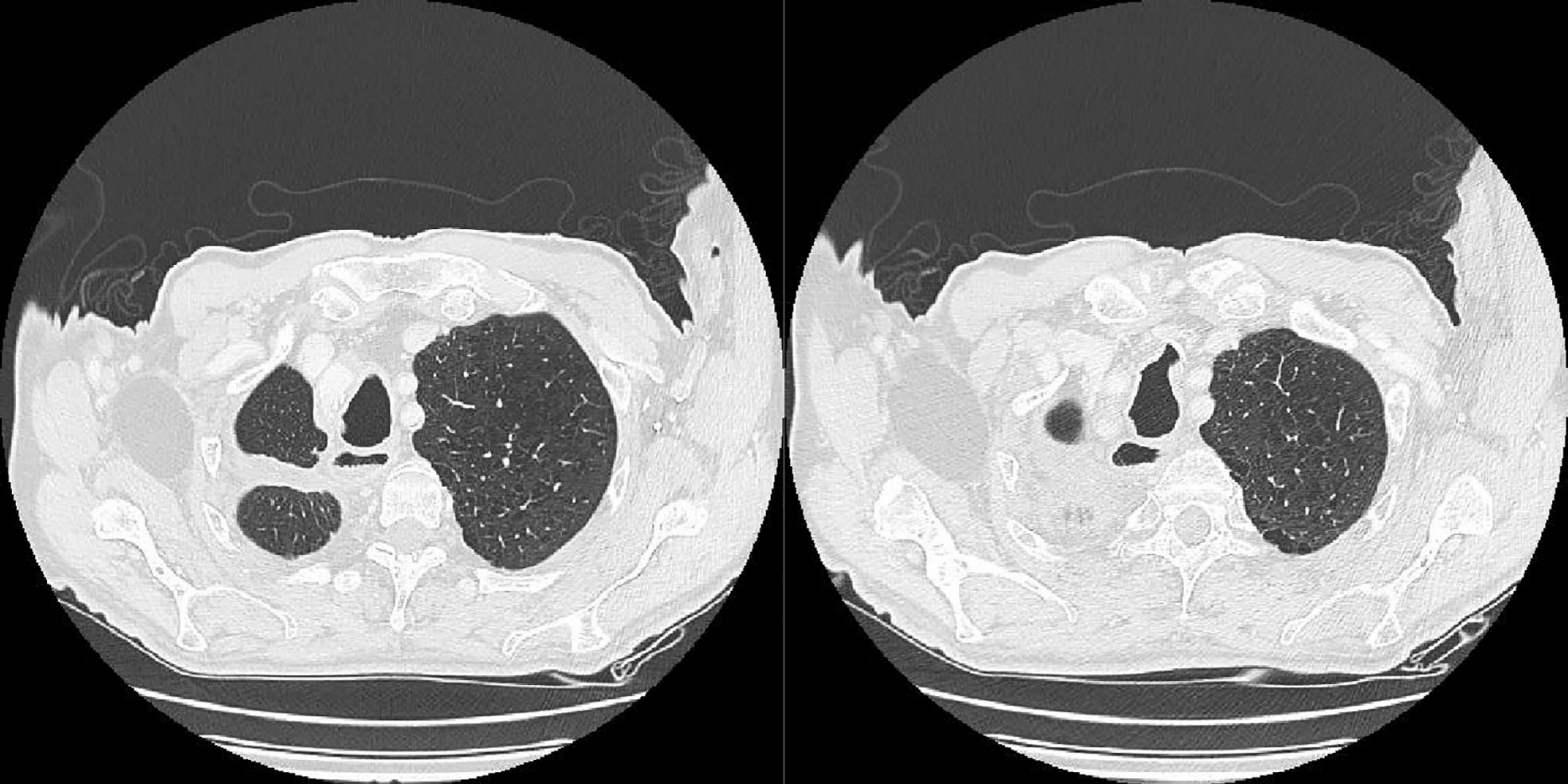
Total body computed tomography scan taken after four years of follow‐up, negative for recurrence or metastatic spread of the primary cancer.
